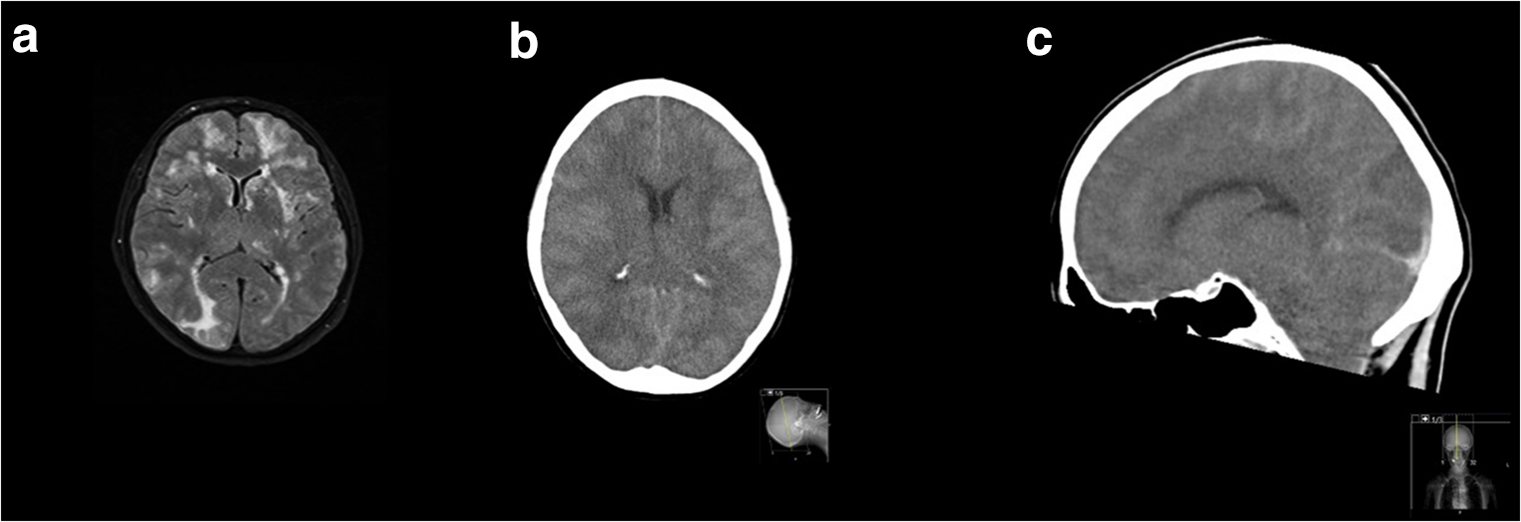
MR and CT imaging of patient 4. MR-T2* image showing diffuse low signal area due to blood products and hyperintense alteration involving predominantly and asymmetrically the frontal, insular and parieto-occipital regions bilaterally, compatible with vasogenic edema (a). CT brain showing diffuse effacement of sulci, consisting with cerebral edema (b, axial scan; c, sagittal view)
